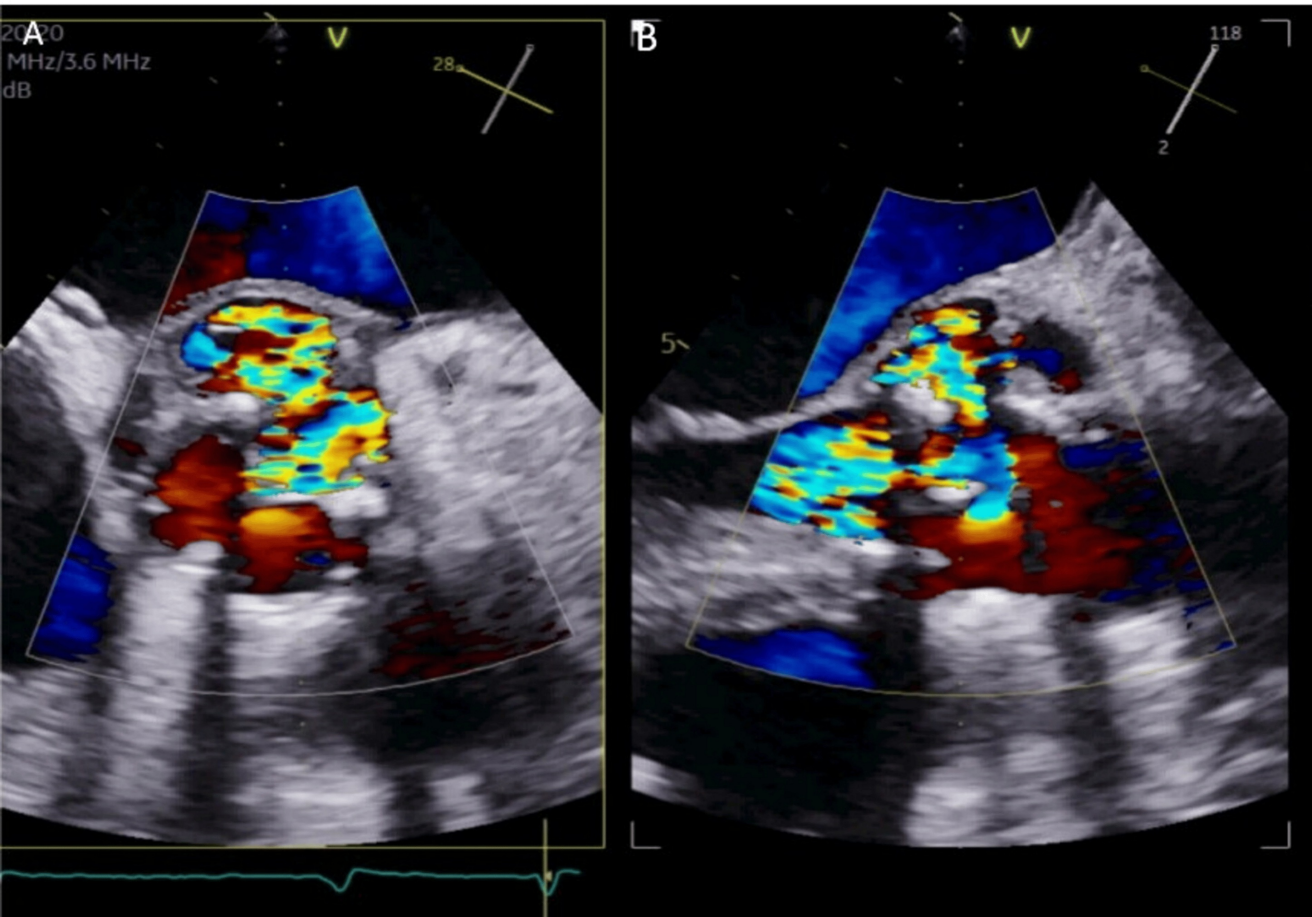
Transesophageal echocardiography showing moderate-to-severe aortic regurgitation
